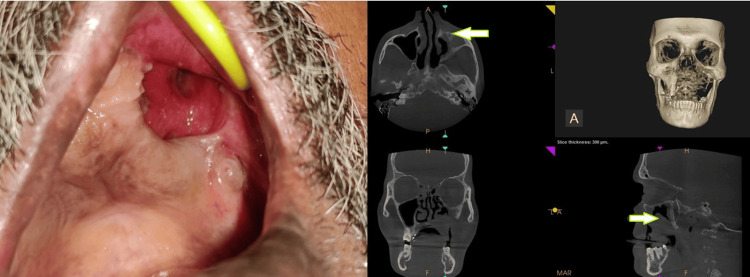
Clinical and radiographic (full skull CBCT) images showing the extent of involvement in mucormycosis. Clinical picture demonstrates the destruction of the maxillary alveolus with the exposure of the underlying tissues. Full skull CBCT image shows osteolytic areas of destruction involving the maxillary alveolus, extending to the maxillary sinus, with the obliteration of the ostiomeatal complex. CBCT: cone-beam computed tomography
